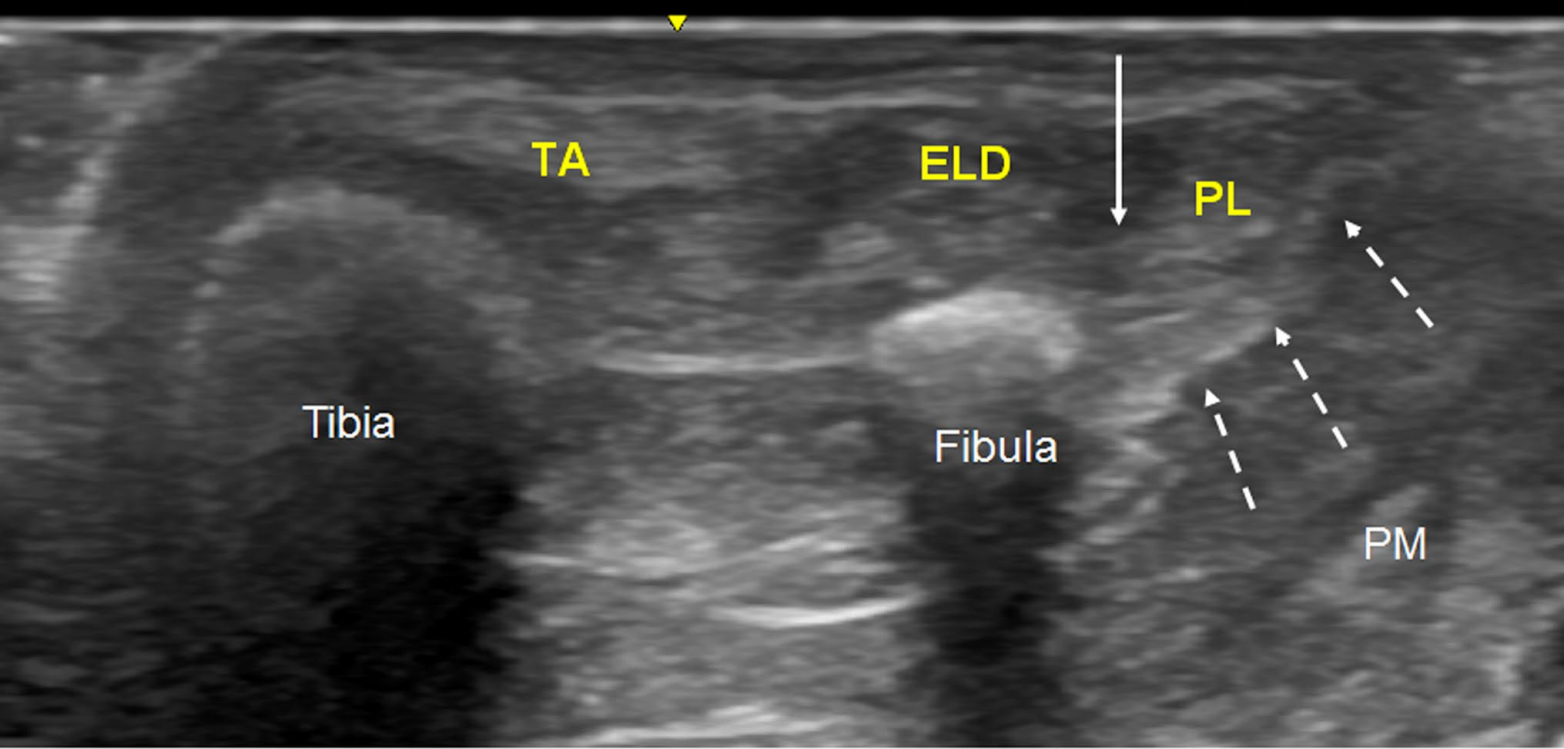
Ultrasound view of the fetus from 29 weeks. There is no septum between tibialis anterior (TA) and extensor digitorum longus (EDL) muscles; however, there is a septum between the EDL and peroneus longus muscle (PL) (white arrow) and PL and posterior compartment (PC) (white broken arrows)
